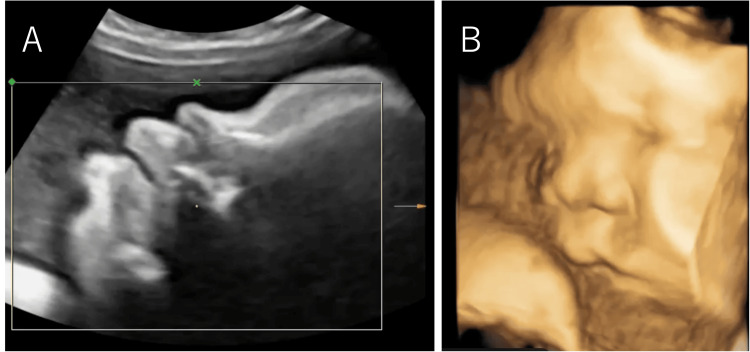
Ultrasound findings showing 2D and 3D images of the fetus’s face. A. Sagittal 2D ultrasound findings did not show any tongue protrusion. B. The 3D image also does not clearly show macroglossia.
